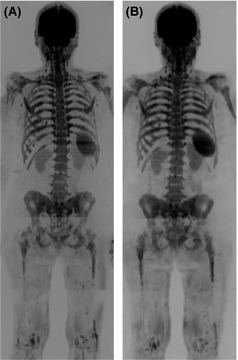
False positive whole body diffusion weighted magnetic resonance imaging (WB DW‐MRI) following granulocyte colony‐stimulating factor (GCSF) administration. b900 WB DW‐MRI Maximum Intensity Projection images in a 67‐year‐old female with relapsed multiple myeloma (A) show diffuse abnormal marrow signal indicating infiltration which was confirmed by 20% plasma cell infiltration on trephine. b900 WB DW‐MRI Maximum Intensity Projection images 6 months later following treatment (B) show stable appearances; however; trephine showed regenerating marrow and no plasma cells. The patients had received GCSF 3 d prior to the MRI (B), which caused a false positive MRI secondary to marrow hypercellularity.
